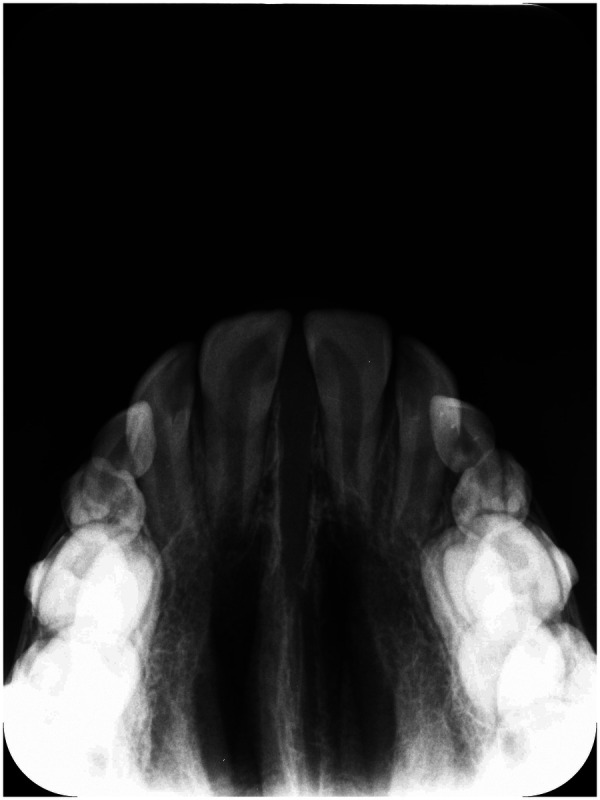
Occlusal radiograph at T1 in a patient treated with IPE.
